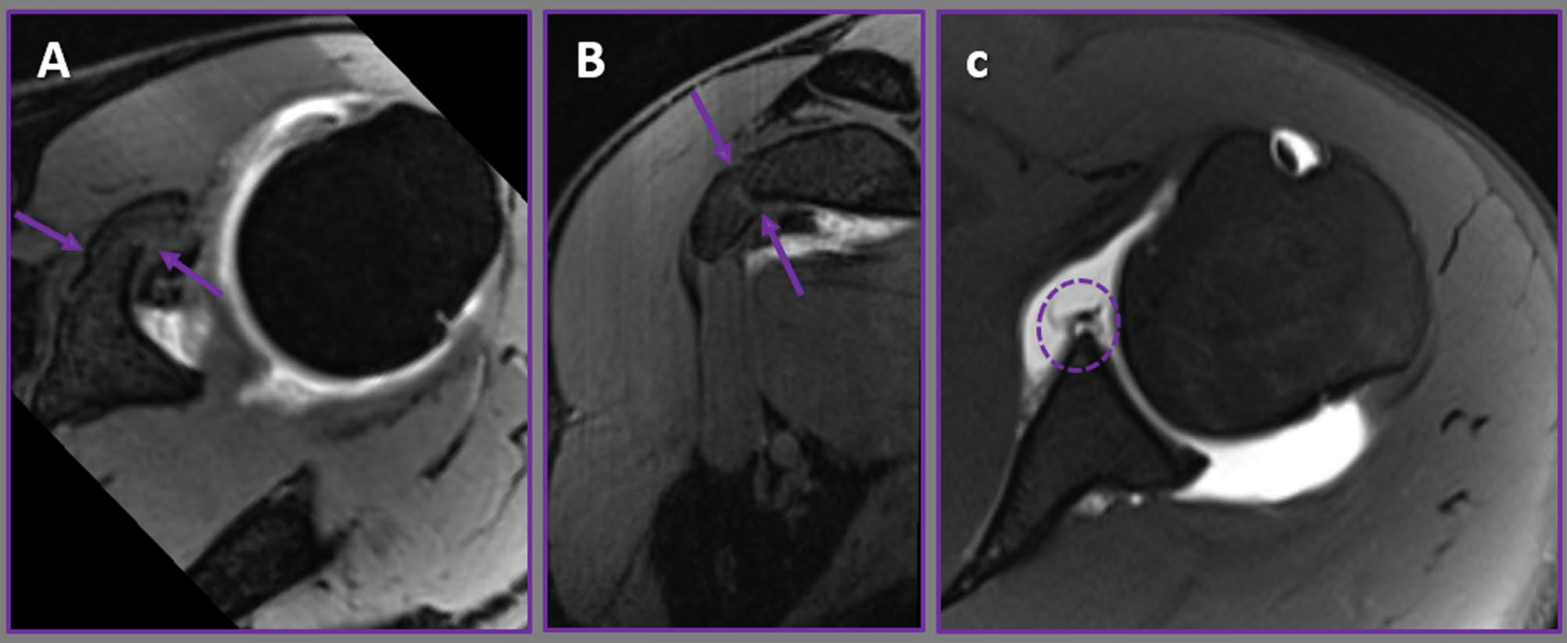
Axial (A) and oblique coronal(B) fat-suppressed T1-weighted VIBE MR arthrograms demonstrate an avulsion fracture (arrows) of the coracoid process. Axial T1-weighted SE (C) MR arthrogram shows associated Bankart lesion (circle)
